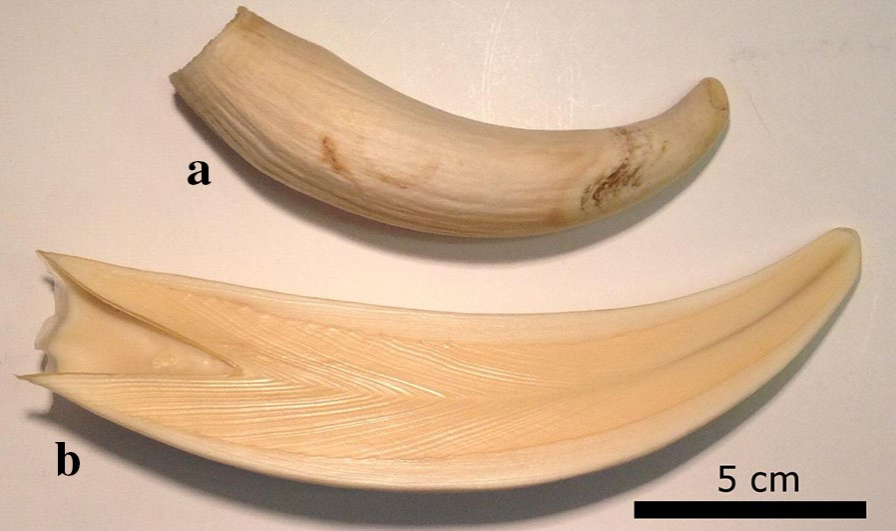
The two whale teeth (tooth a MCE1652 and tooth b MCE1654) used for evaluation of the use of ZTE MRI in whale age estimation
